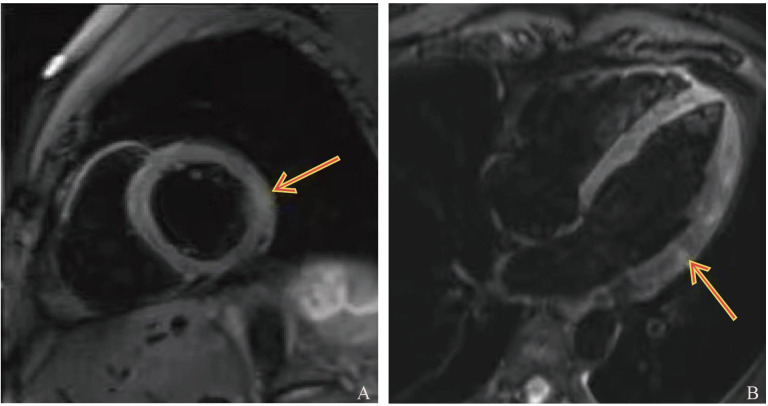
CMR imaging findings of acute myocarditis. (A) Short-axis T2-STIR images revealed segmental hyperintensity in the left ventricular lateral free wall (arrow), consistent with acute interstitial myocardial edema. (B) Long-axis LGE images revealed patchy and linear hyperenhancement (arrow) in the inferolateral wall of the left ventricle, predominately involving the mid-myocardial and subepicardial layers. CMR, cardiac magnetic resonance; LGE, late gadolinium enhancement; T2-STIR, T2-weighted Short Tau Inversion Recovery.
